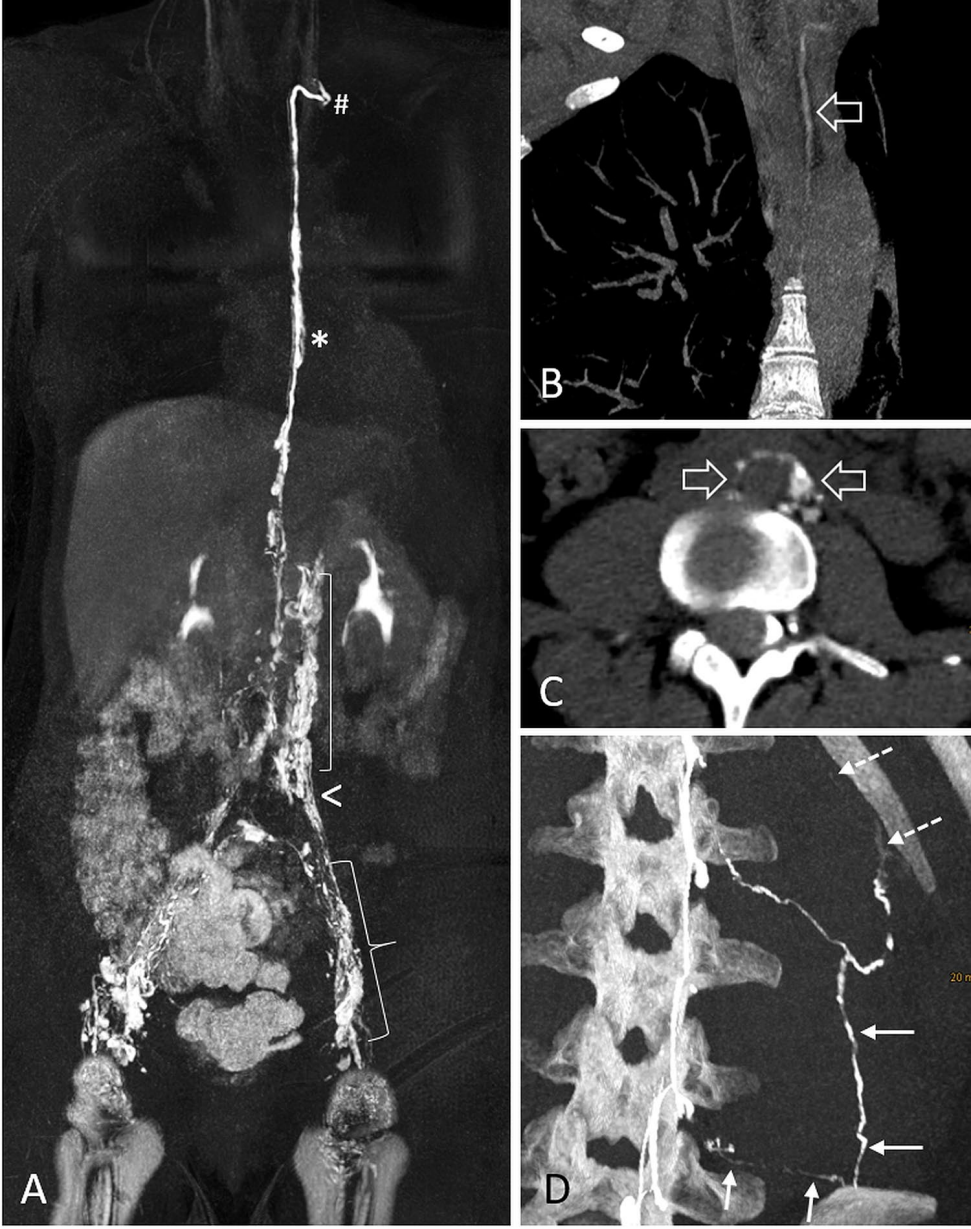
Physiological lymphatic pathways and drainage of the CSF-lymphatic fistula. MR lymphangiography (MRL) visualizing lymphatic drainage pathways from inguinal lymph nodes into iliac (curly bracket in A) and retroperitoneal lymphatics (square bracket in A), further into the lymphatic duct (asterisks in A) and finally into the left lymphatic-venous junction (rhomb in A), excluding an underlying lymphatic malformation. White arrowhead in A indicates the level at which the CLF entering the retroperitoneal lymphatic vessels. CT myelography (CTM) also showing contrast drainage into the left thoracic duct (open arrow in B) and para-aortic lymphatic chain outlining the aorta (open arrows in C). On the other hand, lateral drainage via the left abdominal trunk into lumbar paravertebral (solid arrows in D) and intercostal lymphatics is visible (dashed arrows in D)
